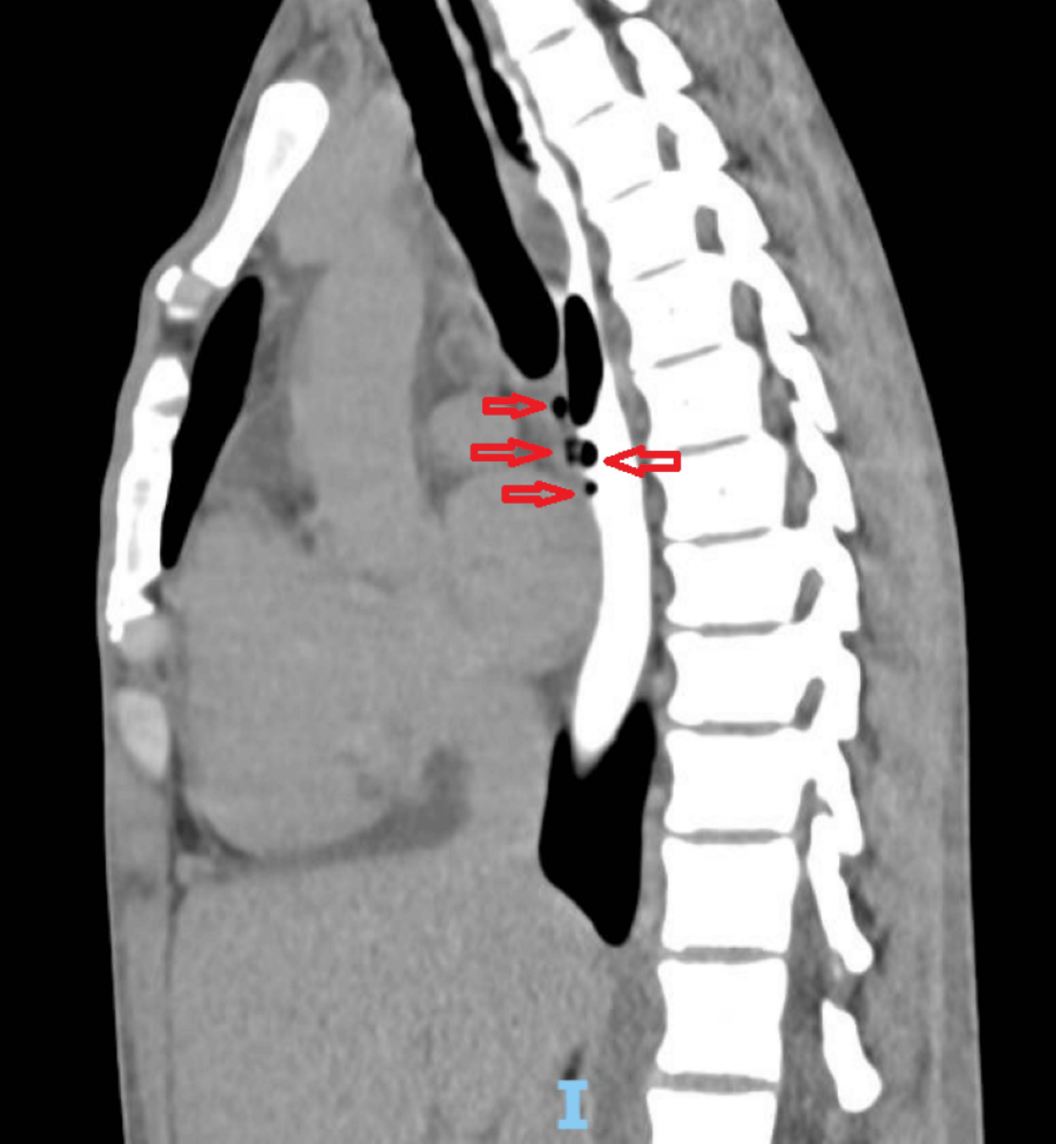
Chest CT scan with double contrast (intravenous and oral) demonstrating, at the middle third of the esophagus, the presence of gas bubbles projecting from the esophageal lumen toward the anterior wall (red arrows), suggestive of a possible fistulous tract following oral contrast administration CT: computed tomography
